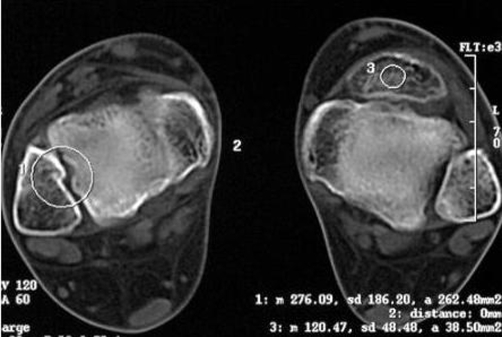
Computed tomography transverse plane of the left ankle was not shown any fracture or osseous pathology.
